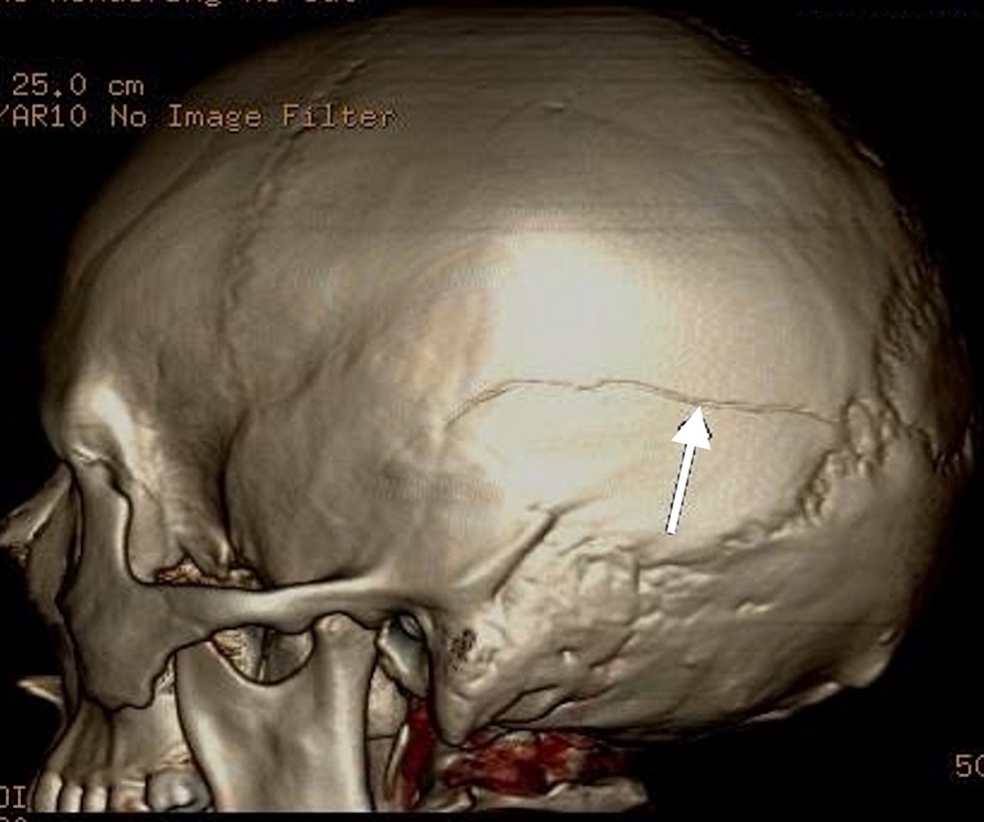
CTA head/neck. A left nondisplaced fracture (indicated by the arrow) in the confluence of the parietal and temporal bones was visualized. CTA: computed tomography angiography.
